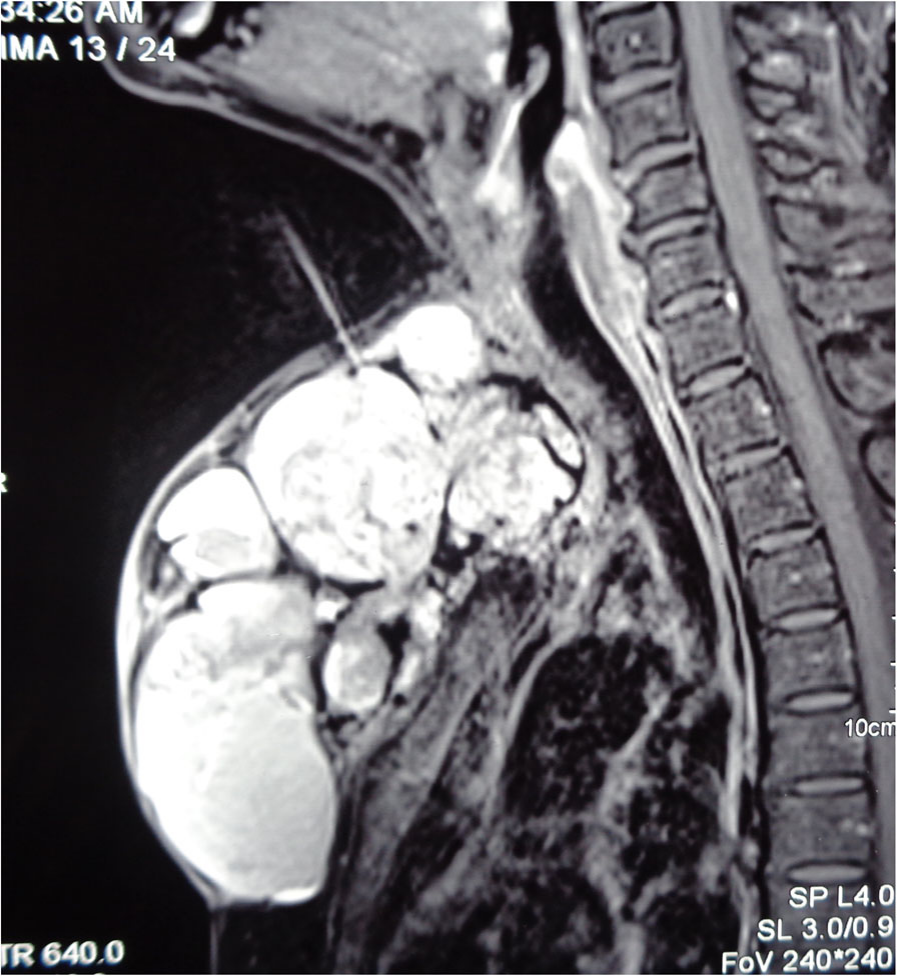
MRI sagittal view- multicystic lesion
